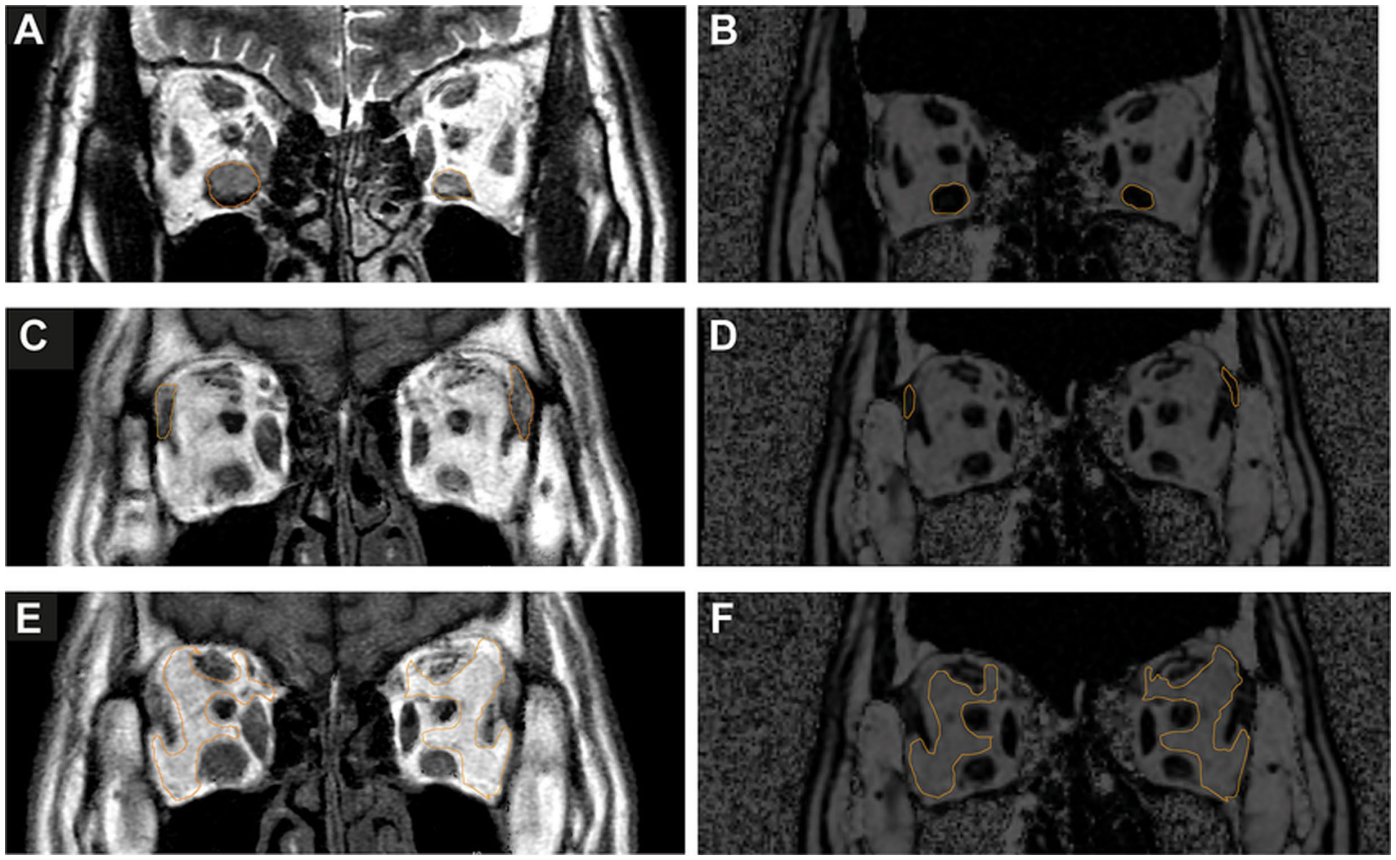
Region-of-interest (ROI) definition for quantitative MRI analysis. The ROI was placed along the perimeter of the greatest enlargement of IR, LG, and OF in a coronal section. (A) IR ROI on MAGiC. (B) IR ROI on IDEAL-IQ. (C) LG ROI on MAGiC. (D) LG ROI on IDEAL-IQ. (E) OF ROI on MAGiC. (F) OF ROI on IDEAL-IQ.
